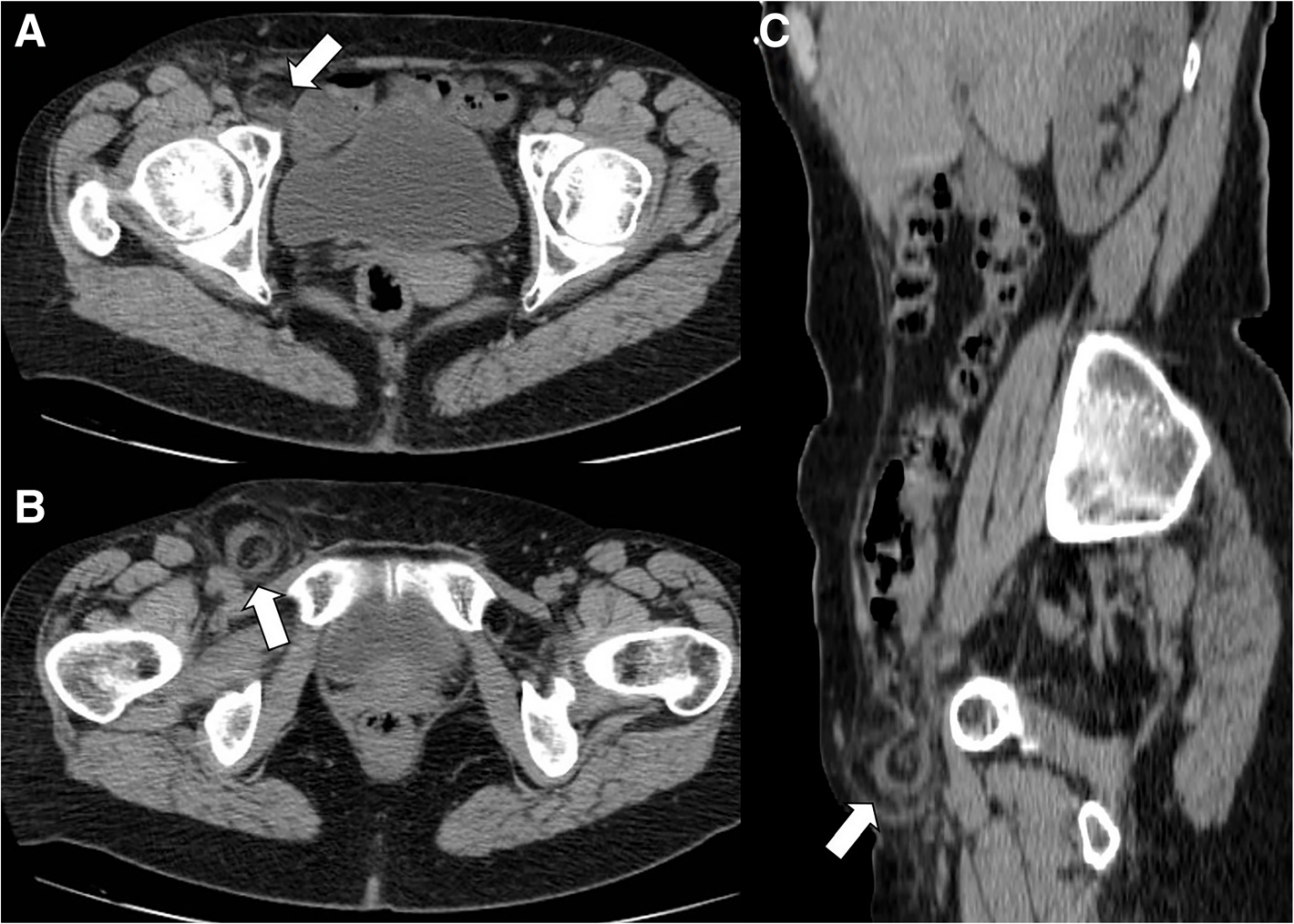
Acute epiploic appendagitis in a 59-year-old woman. Axial non-contrast CT images at two different levels (a, b) and sagittal reformatted image (c) show a right inguinal hernia containing an epiploic appendage surrounded by a thin hyperdense rim (arrows). Fat stranding is seen within the hernia sac. Surgical hernia repair and pathological examination confirmed the presence of an epiploic appendagitis incarcerated in inguinal hernia
